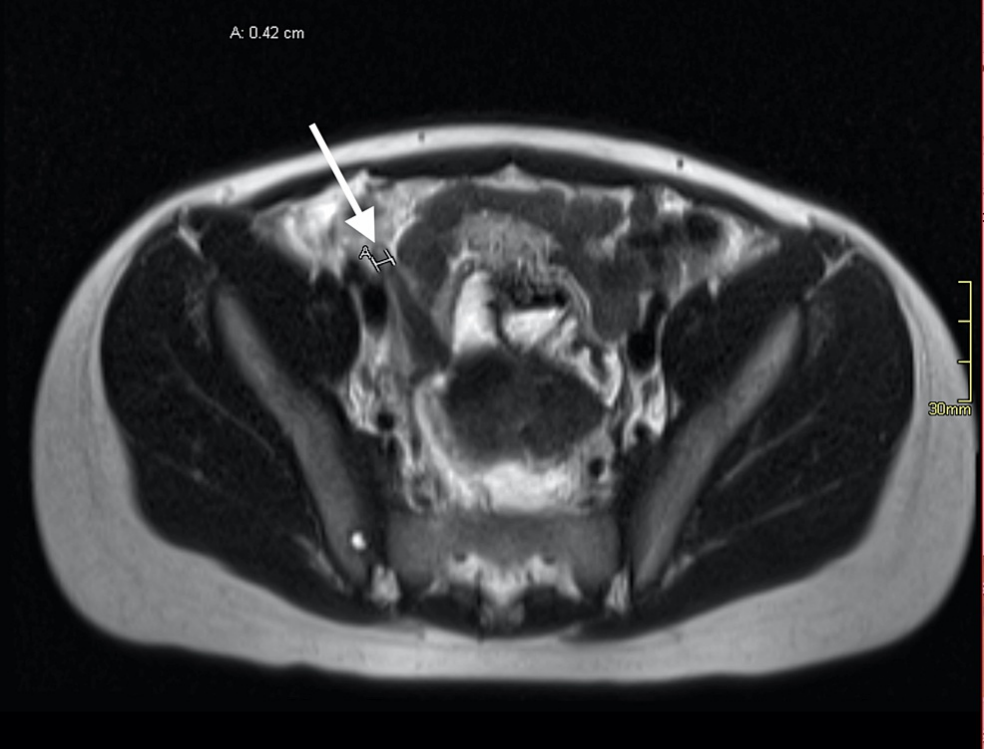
MRI of the abdomen revealing free fluid throughout the peritoneum, retroperitoneum, and extraperitoneal pelvis.
